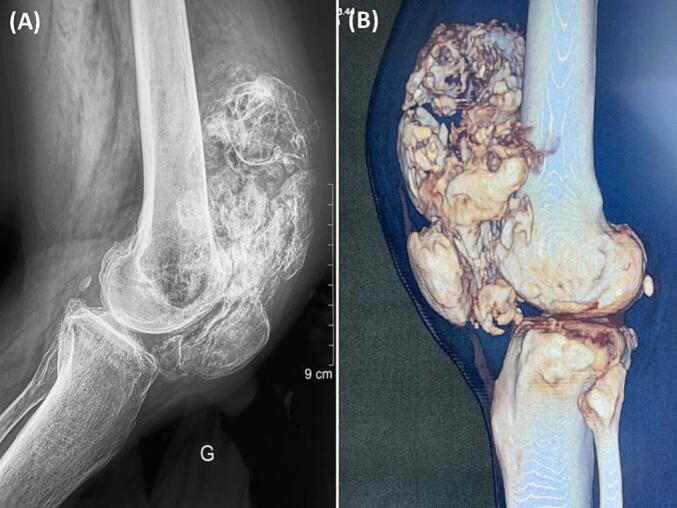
A: Lateral plain X-ray of the left knee revealing an irregular calcific mass extending from below the patella to the suprapatellar region. B: 3D CT scan sagittal view showing multiple loose bodies in the patellofemoral and tibiofemoral joints.
